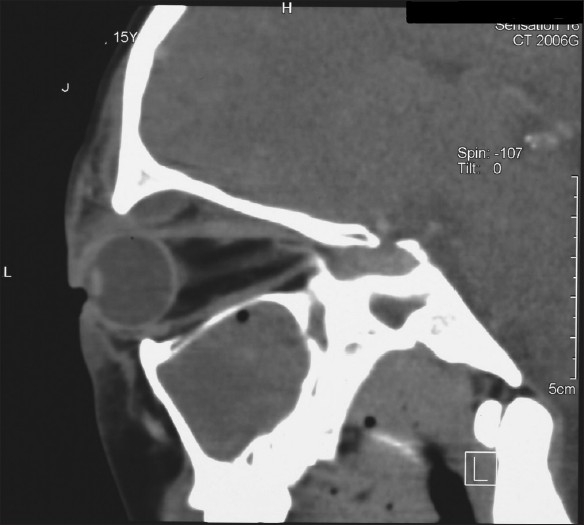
Sagittal computed tomography scan with contrast through the left orbit showing superior abscess and proptosis
